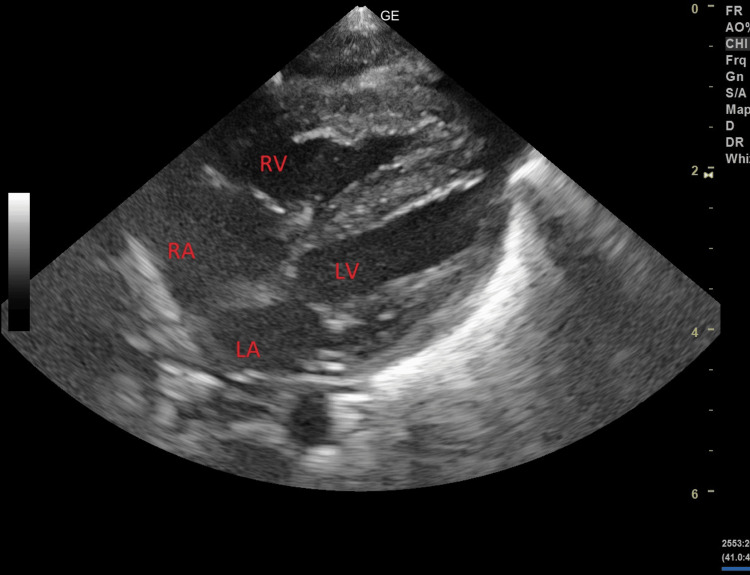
2D echocardiography, subcostal four-chamber view, showing biventricular and septal hypertrophy LA: Left atrium; LV: Left ventricle; RA: Right atrium; RV: Right ventricle
